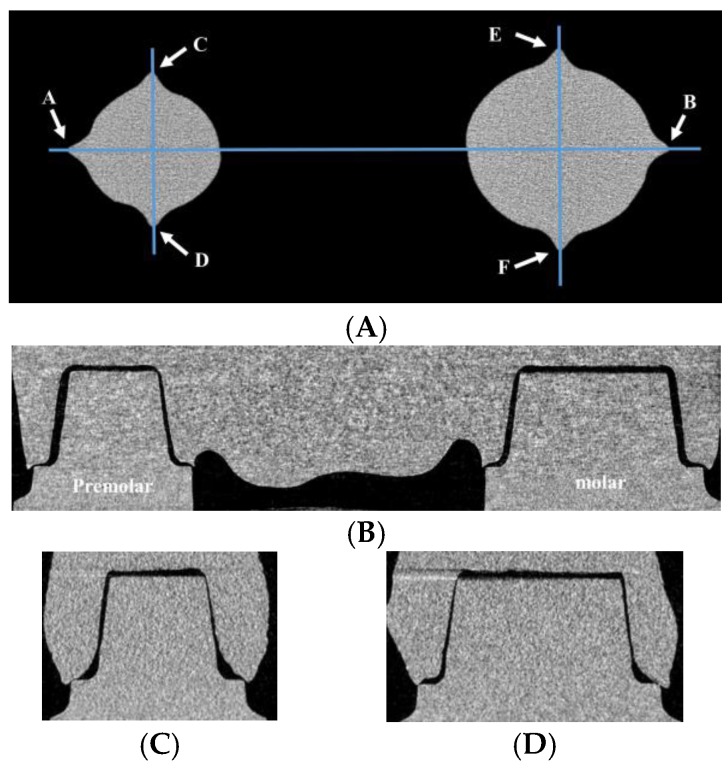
(A) A horizontal plane containing reference points A to F on CT data was defined as a reference plane. On the reference plane, a blue line containing reference points A and B indicates the coronal section. Another line containing reference points C and D indicates the premolar sagittal section and the other line containing reference points E and F indicates the molar sagittal section. (B) The coronal section, (C) the premolar sagittal section and (D) the molar sagittal section.
